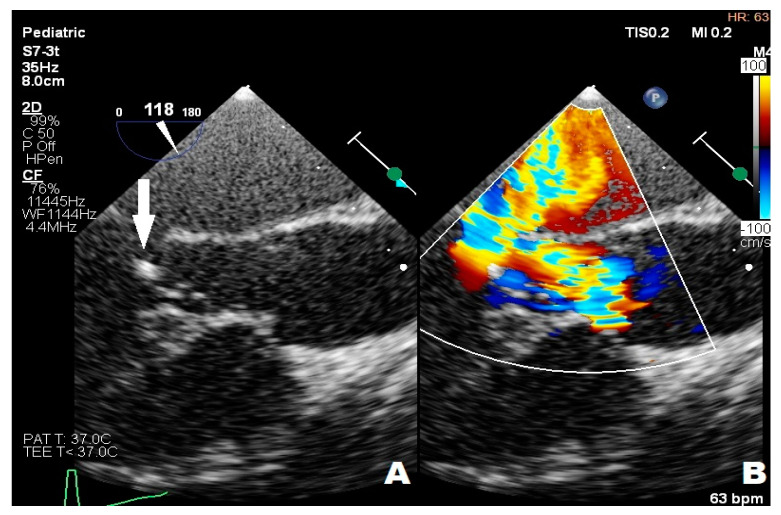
Transesophageal echocardiography; (A) the arrow point at vegetation; (B) mitral regurgitation wave, aortic regurgitation wave.
